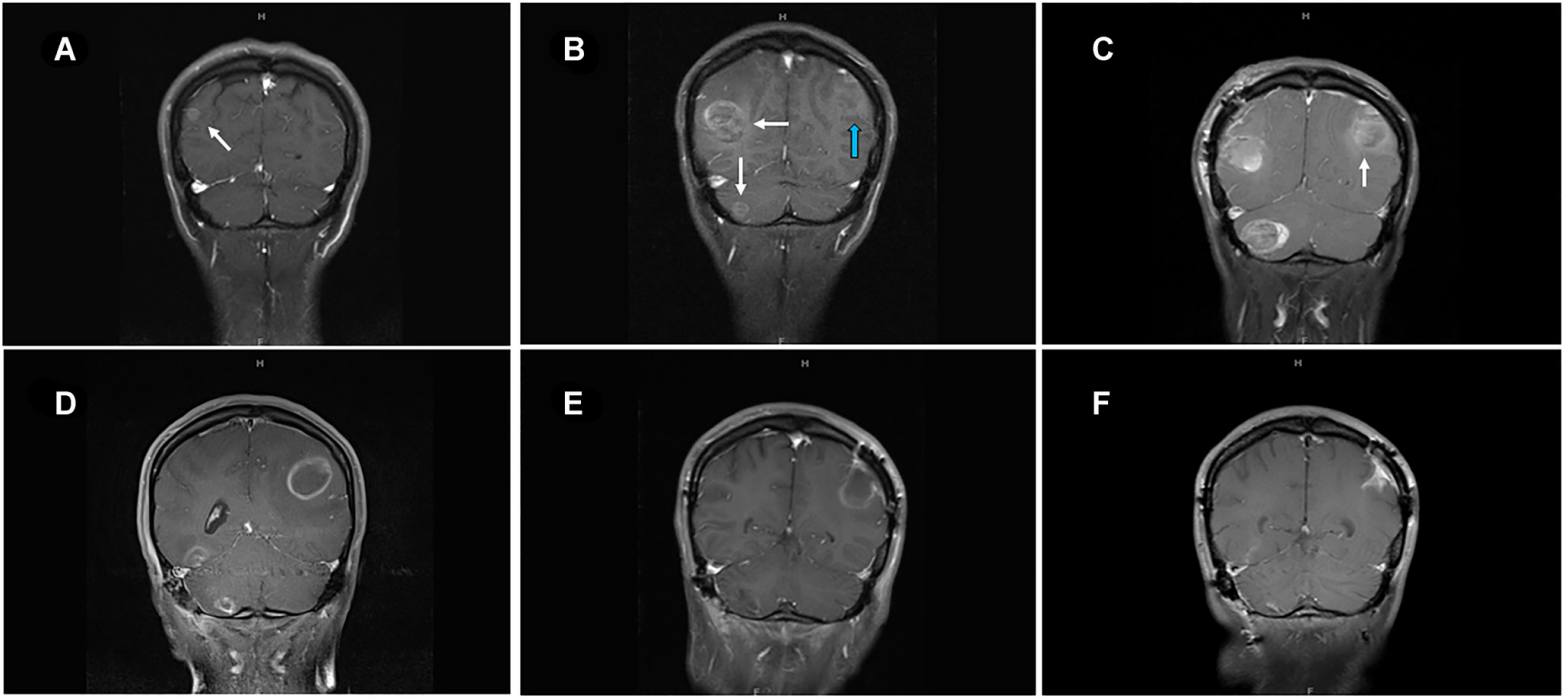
MRI of brain of metastatic lesions showing (A) coronal section demonstrating right parieto-occipital lesion at the start of ipilimumab-nivolumab (arrow). (B) coronal image showing right parieto-occipital and right cerebellar lesions (white arrows) and left posterior parietal lesion (blue arrow) after three cycles of ipilimumab-nivolumab. (C) Post-surgical resection of two symptomatic lesions- right parieto-occipital and right cerebellar lesions with persistent left posterior parietal lesion (arrow). (D) Enlarging left posterior parietal lesion post TVEC and pembrolizumab. (E) Surgical cavity after resection of left posterior parietal lesion and one month after TMZ treatment. (F) Collapsing surgical cavity of left posterior parietal lesion resection with no evidence of recurrent disease in the brain after 19 months of temozolomide initiation.
